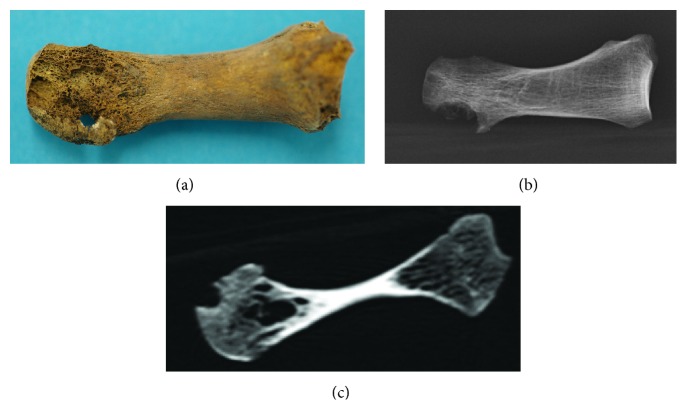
Severe osteolysis of the metatarsophalangeal joint in Gregorius Lechner indicating severe chronic gout (a: macroscopy; b: X-ray; c: CAT-scan).
